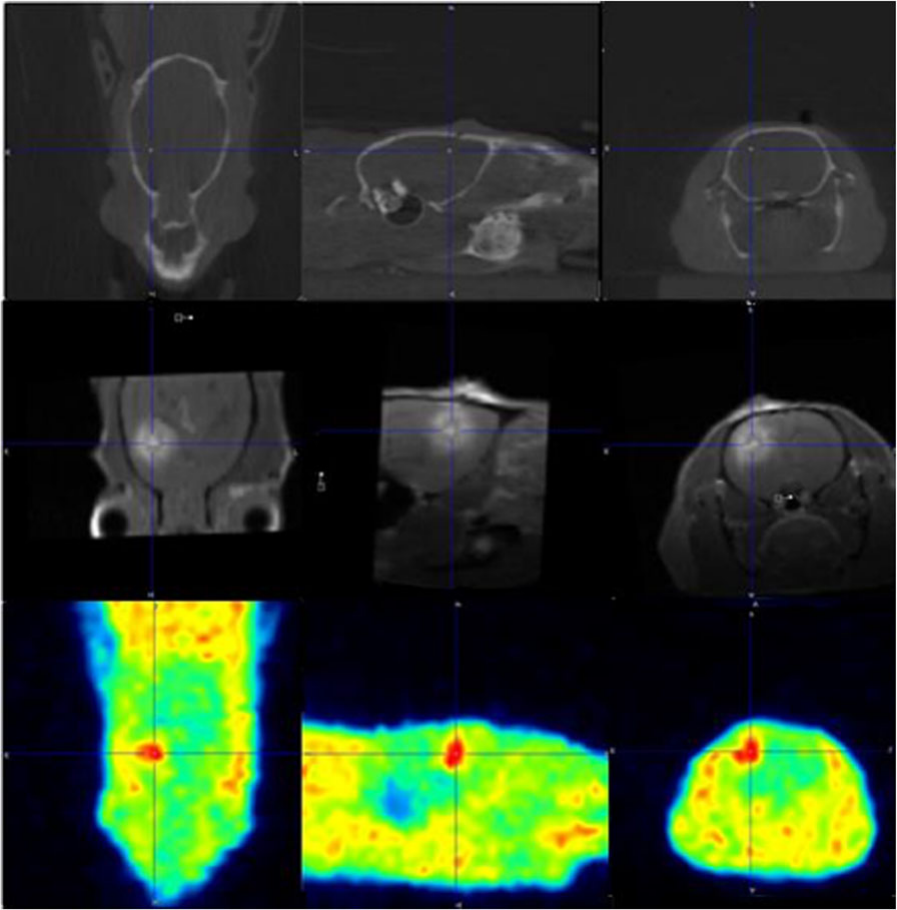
Planning CT (upper row), contrast-enhanced T1w MRI (middle row) and [^18^F]FET PET image. To perform PET-based sub-volume boosting, these three imaging modalities were co-registered
